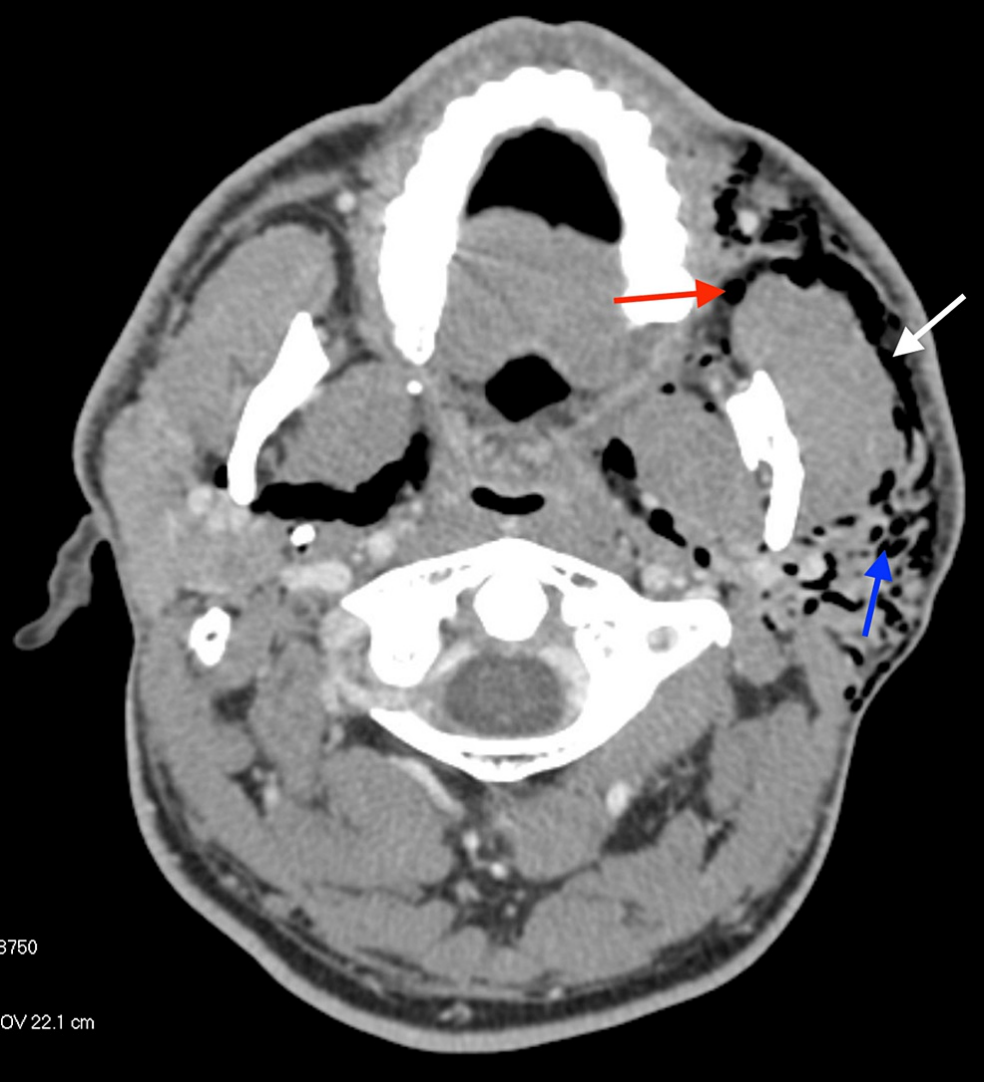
CT Maxillofacial with contrast, axial view CT Maxillofacial with contrast demonstrating air within the parotid gland extending subcutaneously through the soft tissues of the face and along the course of the parotid duct. Red arrow: air around Stensen’s duct. Blue arrow: air within parotid. White arrow: subcutaneous emphysema within soft tissues of face
